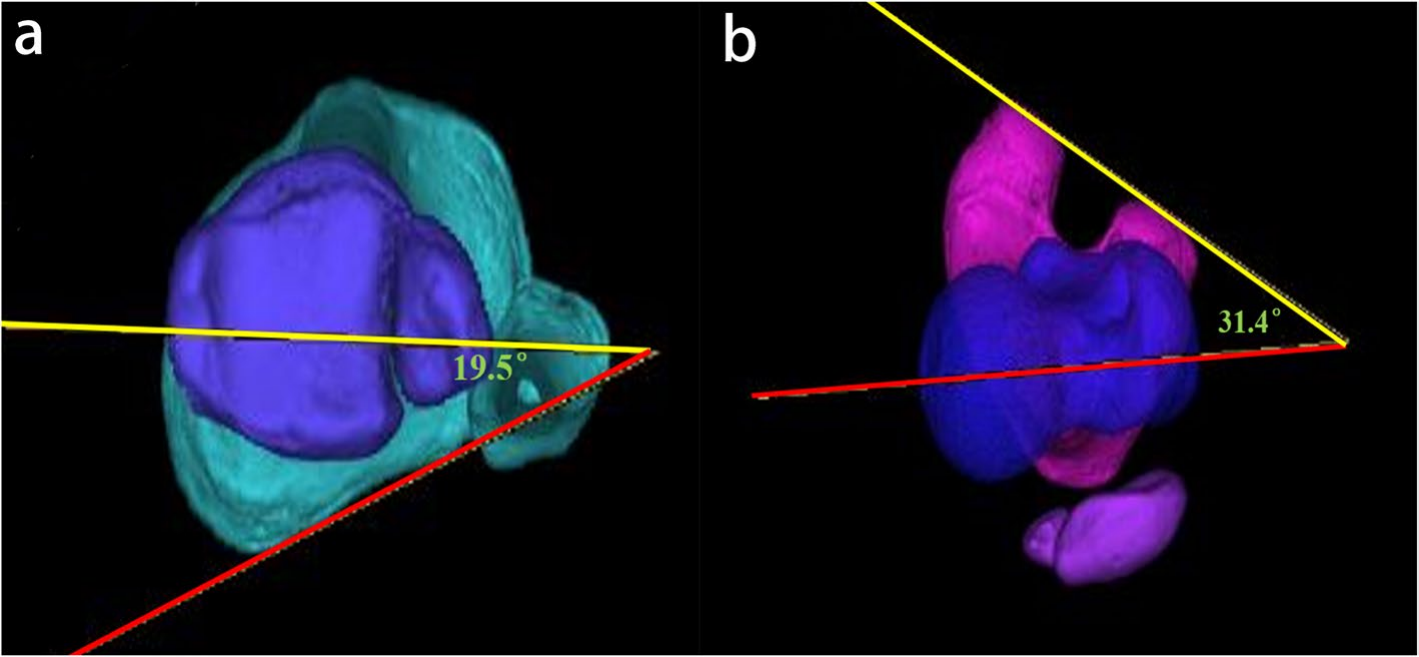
Measurement of torsional parameters of the lower extremity on 3-dimensional computed tomography. a. The tibia external rotation was defined as the angle between the line connecting the posterior tibial condyles and the line connecting the midpoints of the medial and lateral malleoli. b. The femoral anteversion angle was defined as the angle between femoral neck axis (red line) and posterior condylar of distal femoral axis (yellow line)
